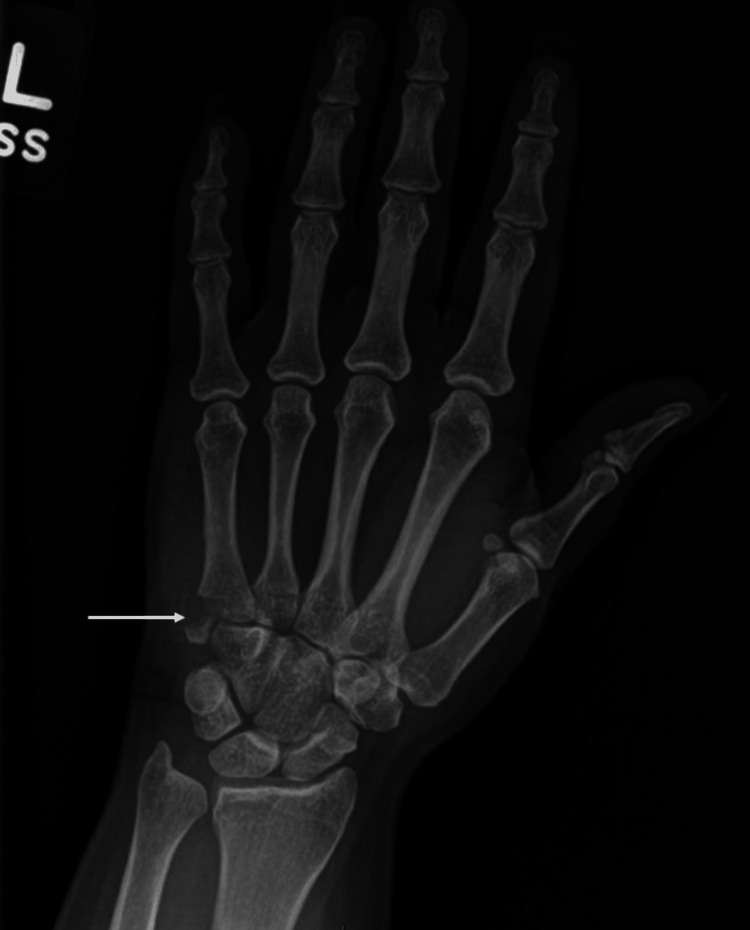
Pre-operative PA radiograph of the left hand.
